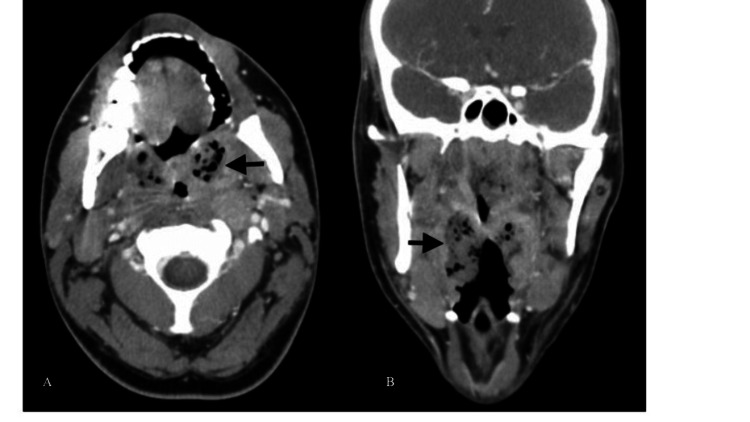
Head CT revealing soft-tissue emphysema in the tonsillar region. A) Axial view and B) coronal view CT, computed tomography.
